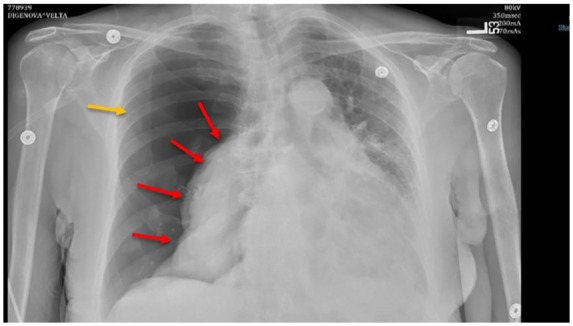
Chest X-ray showing extensive right side pneumothorax (yellow arrow) complicated by complete right lung collapse (red arrows).
